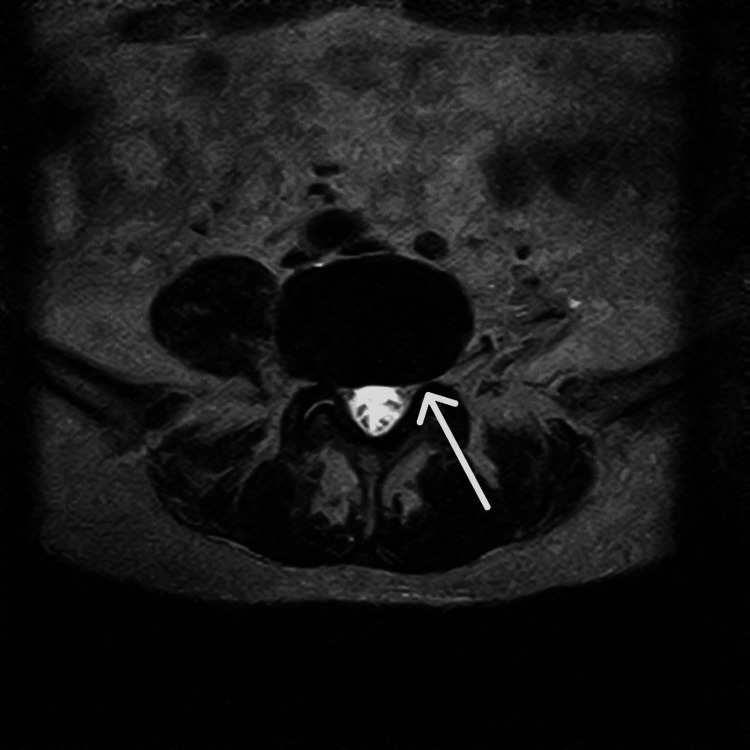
Axial MRI at the L4/5 level showing mild stenosis in the left L5 foraminal region
